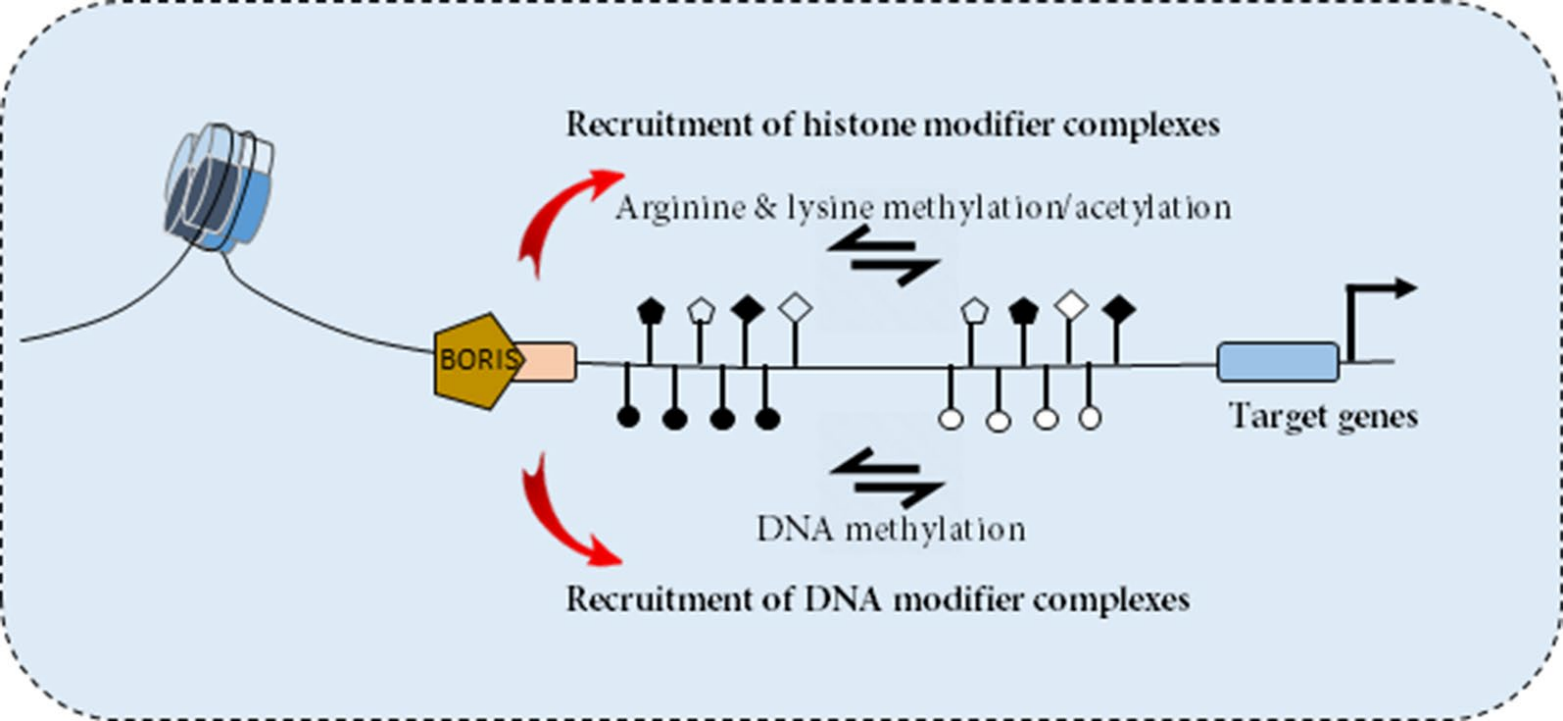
Role of BORIS as an epigenetic modifier regulating the expression of multiple proto-oncogenes and CT genes. BORIS recruits histone modifier complexes to the promoter of target genes to induce tri-methylation of lysine 4 of histone H3 tail, and methylation of arginine and acetylation of lysine 14 of histone H3 tail. BORIS also is involved in promoter methylation/demethylation of target genes. Therefore, BORIS creates a permissive/active chromatin conformation via epigenetic modifications that lead to the upregulation of target genes such as CT genes, Oct4 and Notch3
